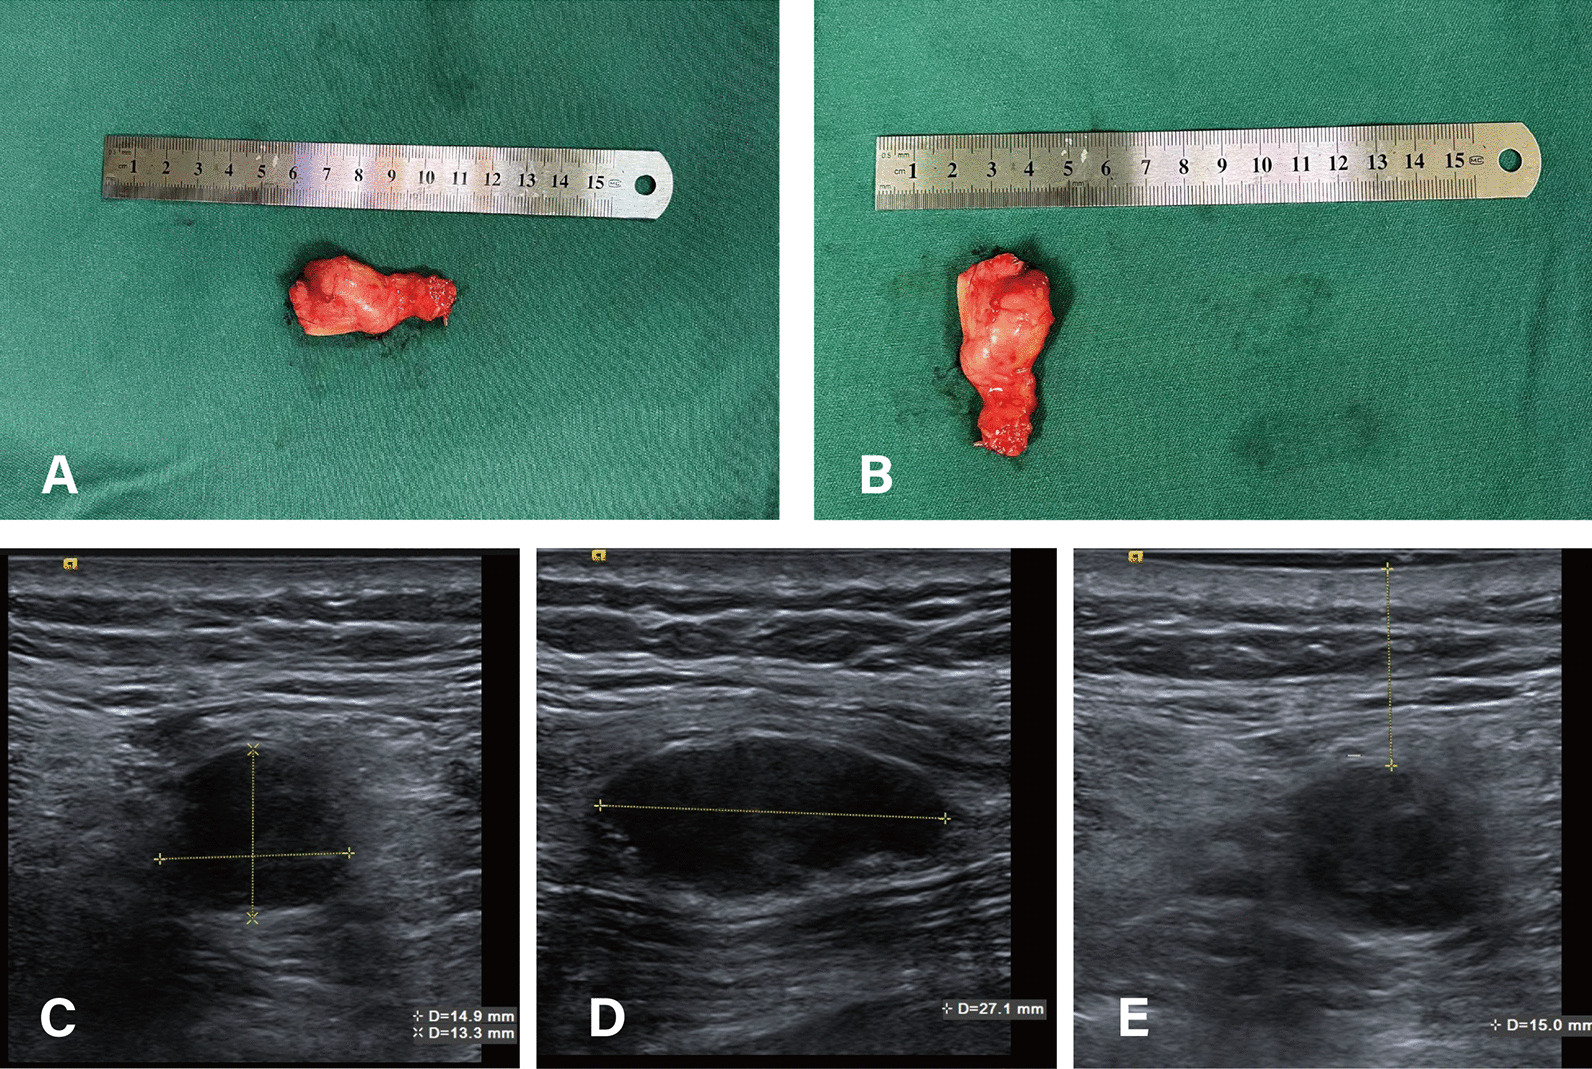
The amputated patient with traumatic neuroma underwent a neuroma ultrasound examination before the surgical resection. A–B The roughly estimated size of the resected neurofibroma by pathological examination after surgery is about 3 × 2 × 1 cm. C–D The ultrasound result indicated that a low-echo nodule with a size of approximately 2.7 × 1.5 × 1.3 cm and clear borders could be seen at the far end of the left sciatic nerve stump. E The depth of the neuroma from the skin was approximately 1.5 cm
